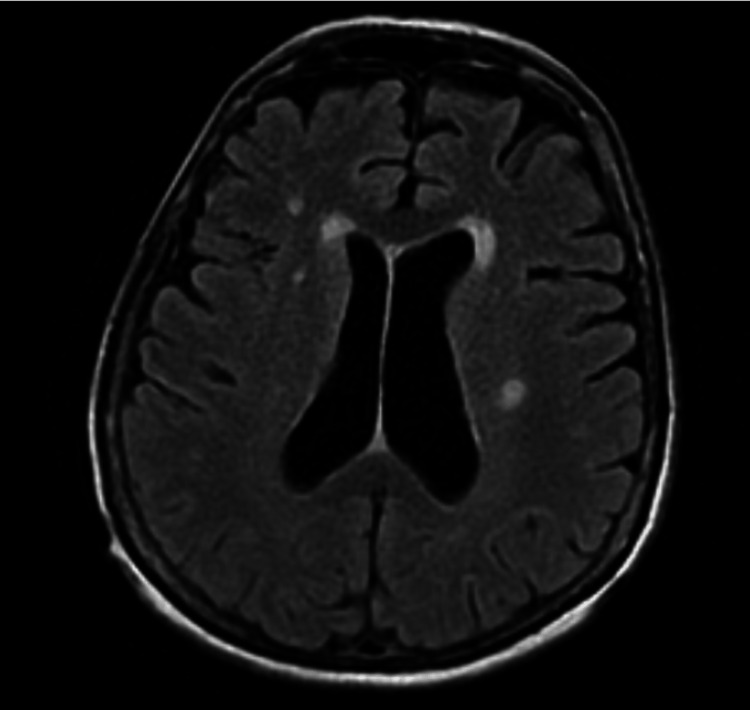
MRI brain of the patient demonstrating an acute stroke to her corona radiata in the setting of her irregular rhythm with conversion pauses and resumption of sinus rhythm
